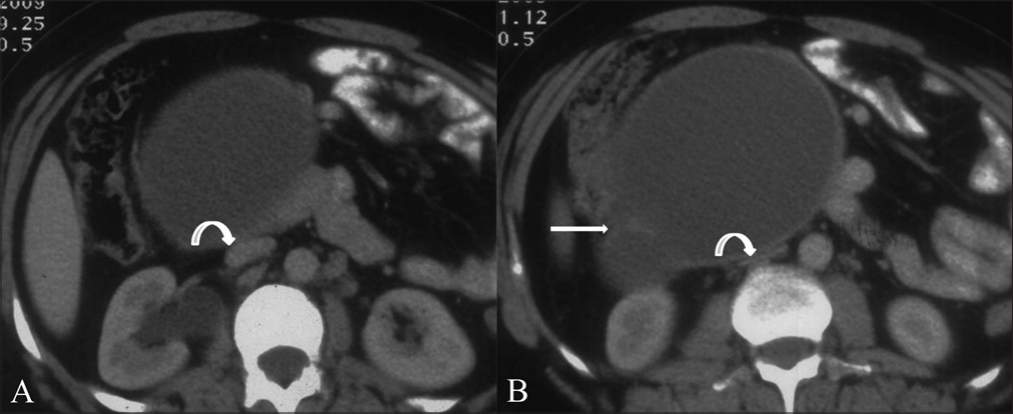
Contrast-enhanced CT scan of the abdomen at the level of inferior pole of the right kidney shows a cystic lesion with internal septae (arrow) anterior to the inferior vena cava (IVC). The IVC is compressed (curved arrow)
